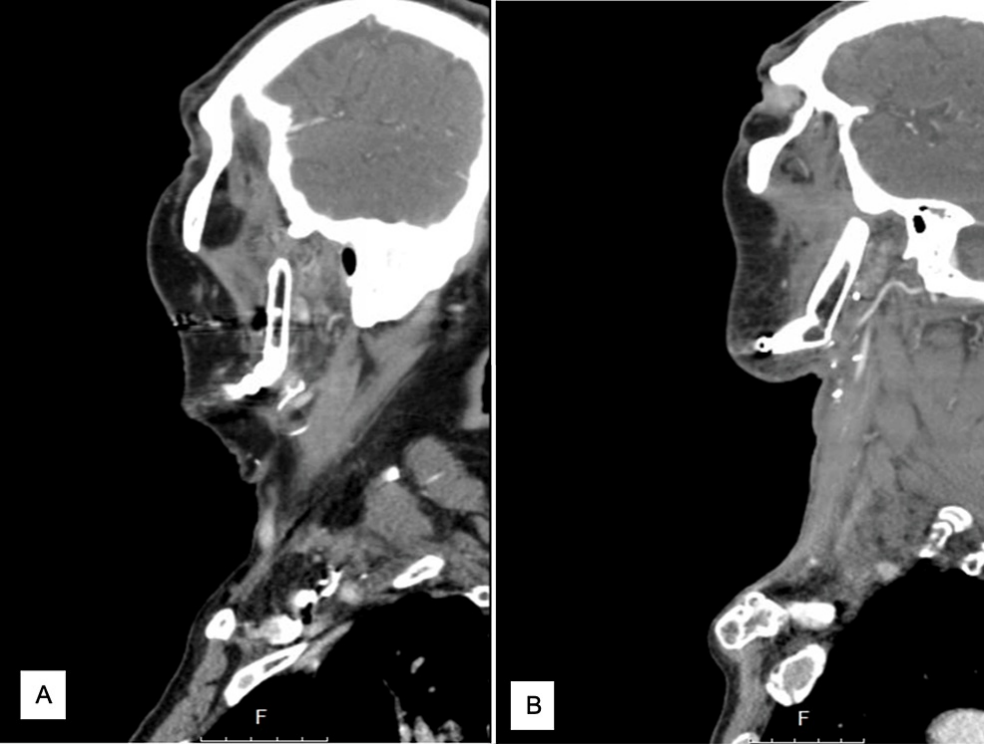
CT imaging of anterior displacement greater than 7.5 mm in two sagittal views (A and B).
